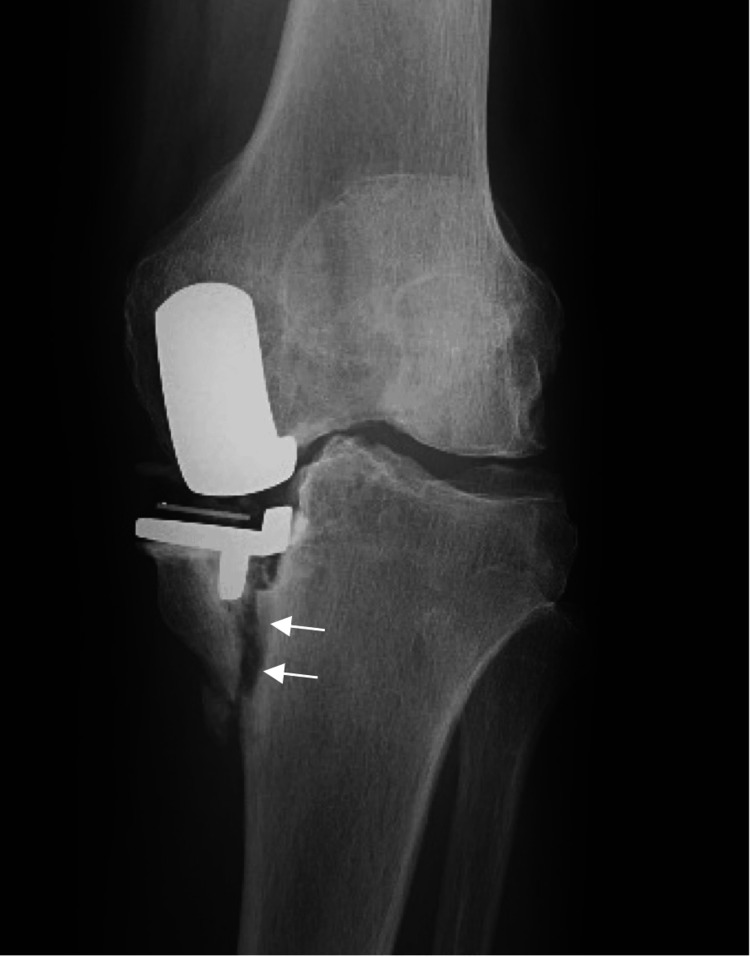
Radiography of the peri-implant fracture
